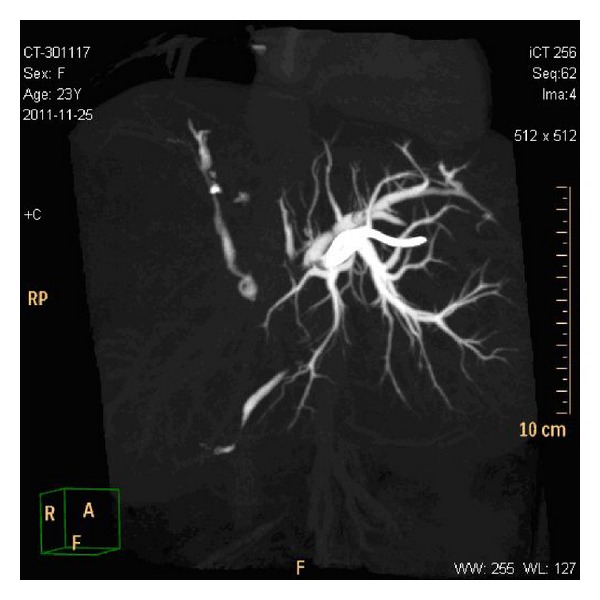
CT cholangiography showed filling defects in the right posterior sectoral duct, common hepatic duct, and CBD.
